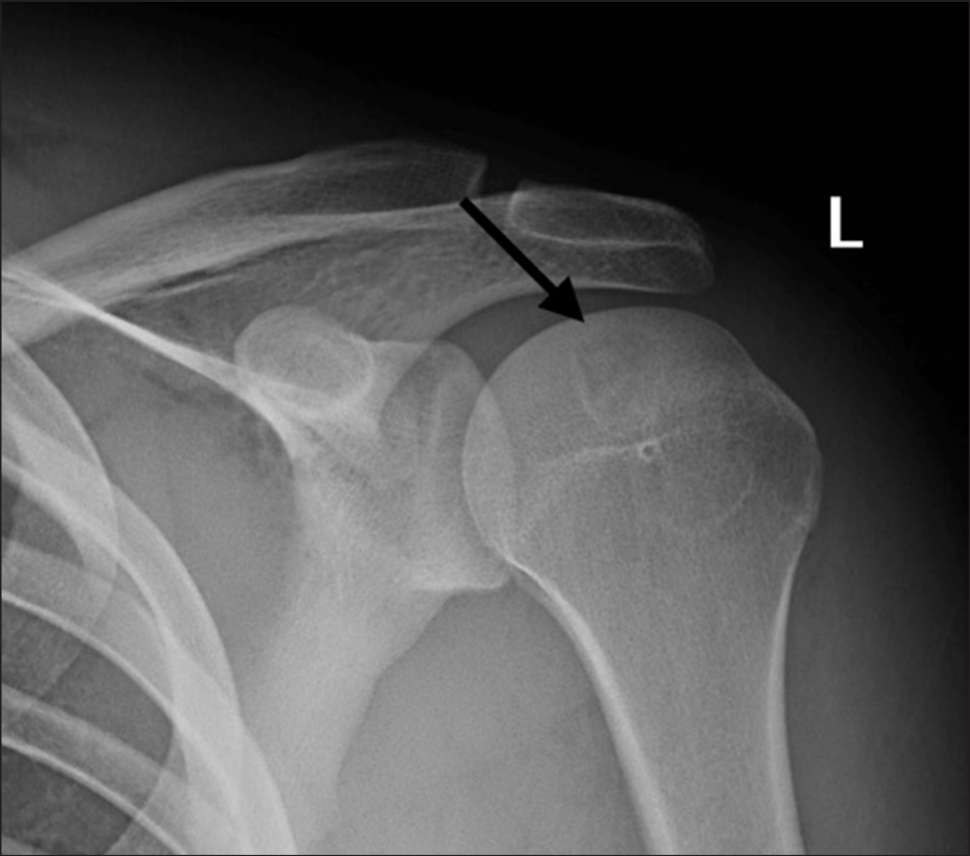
Radiograph of the left shoulder, AP view: a rounded lytic lesion is seen in the epiphysis of the humeral head, with sharp borders and a thin sclerotic rim (arrow).
